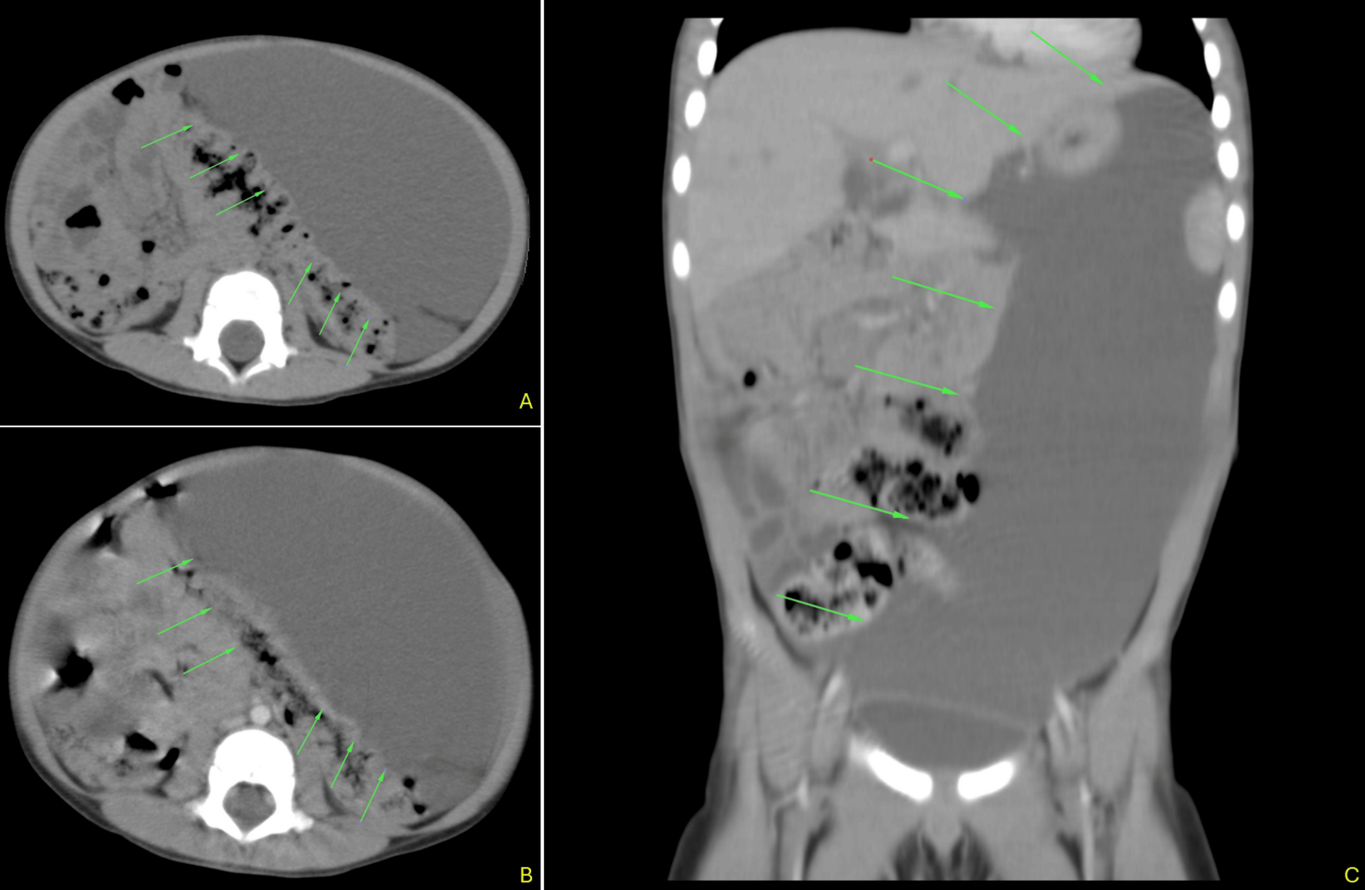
Computed tomography scan images (axial in A and B, coronal in C) demonstrate a massive, homogeneous cystic mass with thin walls in the left hemiabdomen (arrows). The lesion compresses the bowels posteriorly and to the right
